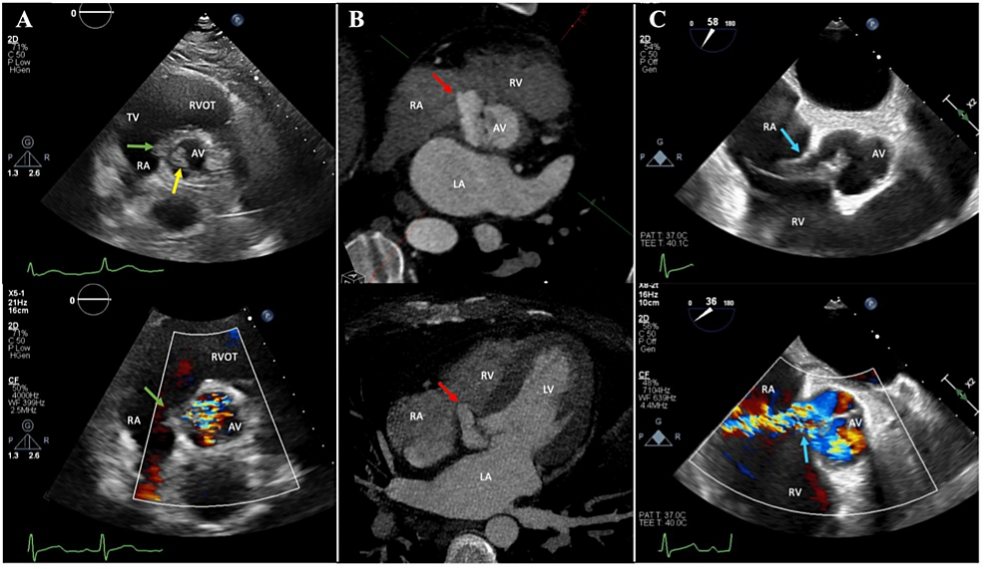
Rapid progression of aortic valve endocarditis. (A) Transthoracic echocardiographic imaging (day 3) shows aortic valve endocarditis (yellow arrow) complicated by severe aortic insufficiency with concern for extension into the aortic root (green arrows). (B) Cardiac computed tomography (day 4) demonstrates pseudoaneurysm of the noncoronary cusp of the aortic valve (red arrows). (C) Transesophageal echocardiogram (day 5) shows rupture of the aortic root pseudoaneurysm with fistula formation between the aortic root and right atrium as demonstrated by color Doppler (blue arrows). Abbreviations: AV, aortic valve; LA, left atrium; LV, left ventricle; RA, right atrium; RV, right ventricle; RVOT, RV outflow tract; TV, tricuspid valve
